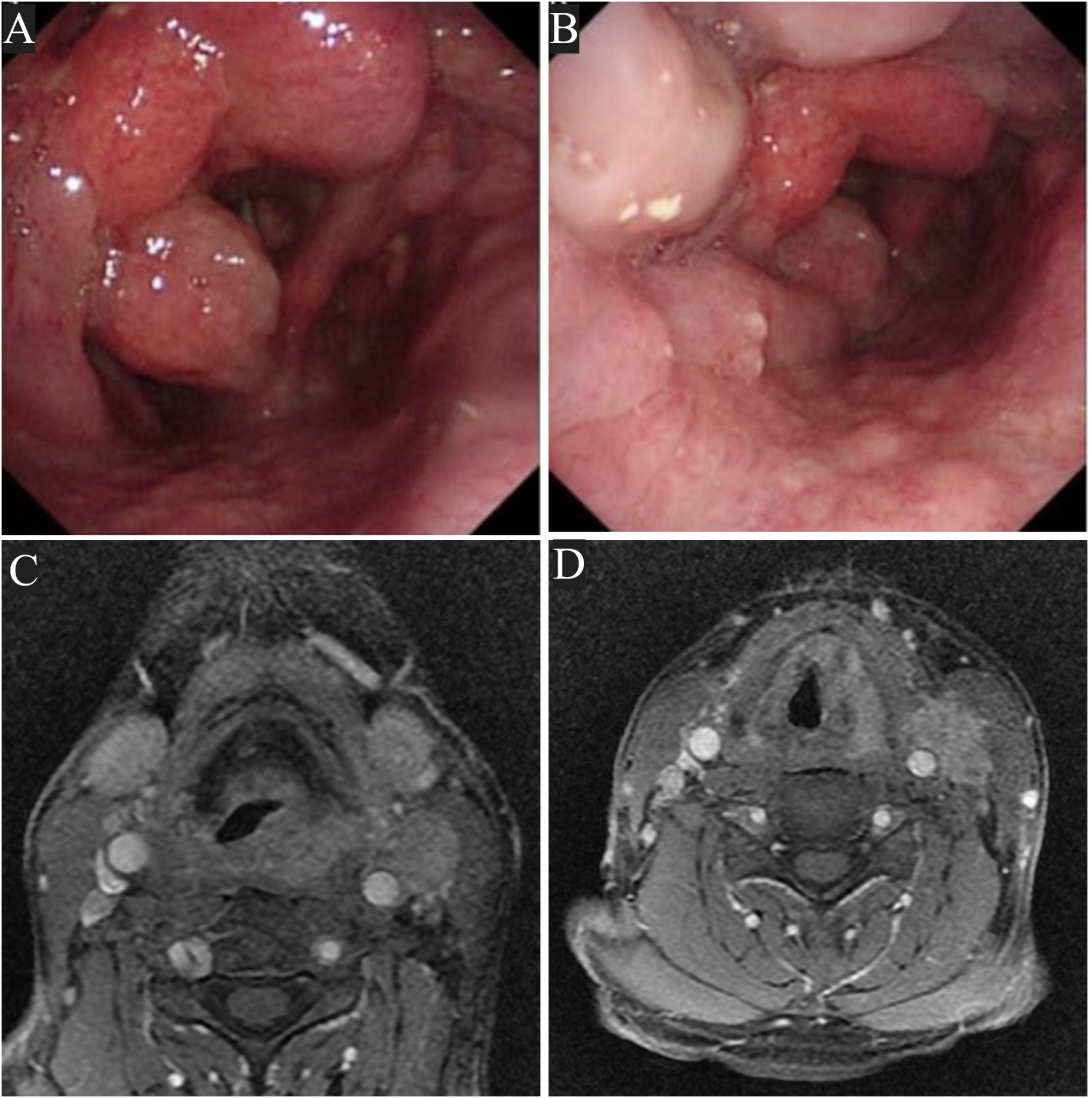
Pre-treatment laryngoscopy (A, B) and contrast-enhanced MRI (C, D). This demonstrated a hypopharyngeal carcinoma with extensive involvement of the left pharyngolaryngeal region, accompanied by bilateral cervical lymph node metastases.
